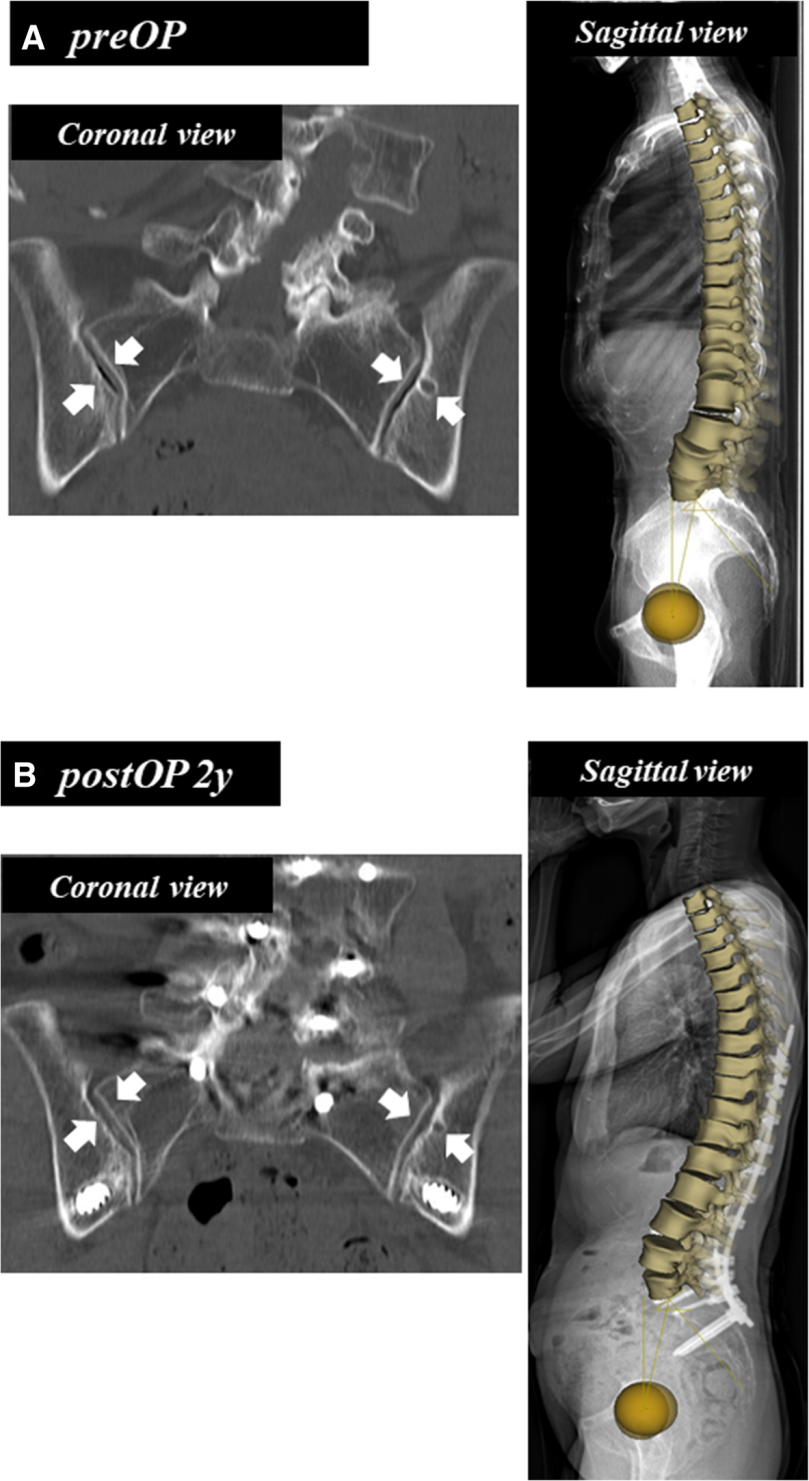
Reconstructive coronal reconstructed CT images of bilateral sacro-iliac joints and corresponding EOS images of the patient (Figs. 2 and 4). a Preoperative images. Arrows indicate vacuum in the bilateral sacro-iliac joints and subchondral cyst. b Images postoperative 2 years. Arrows indicate vanished vacuum in the joints and diminished subchondral cyst
